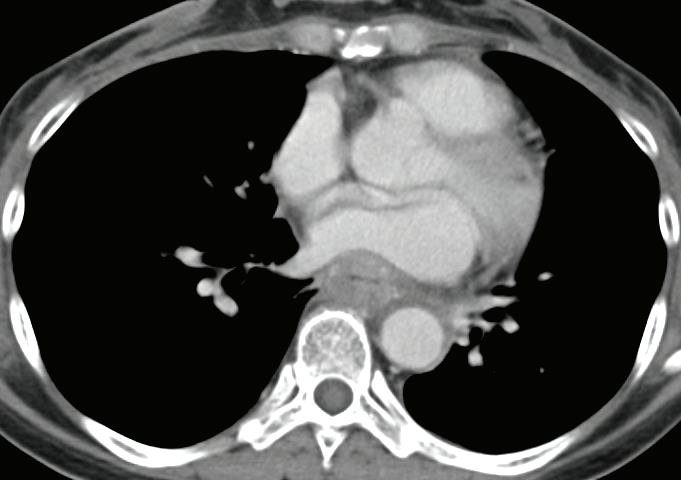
CT image. An esophageal primary esophageal tumor is compressing the left atrium, but direct invasion is not evident.
